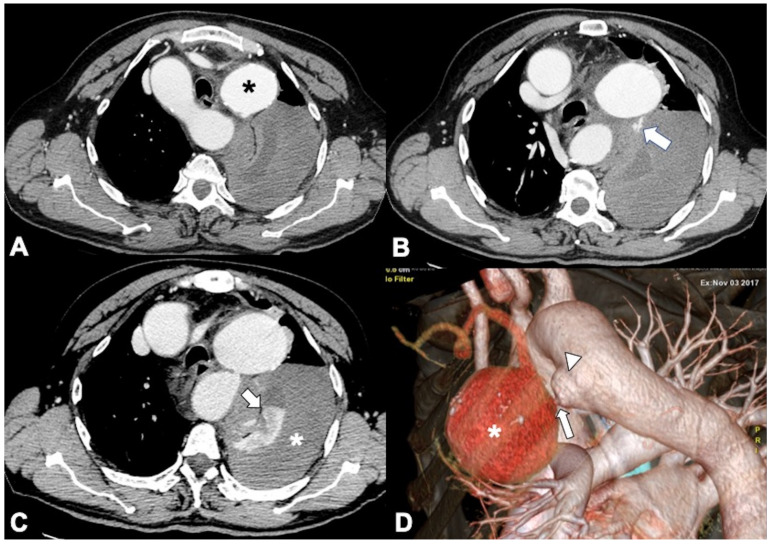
Computed tomography angiography (CTA) provides a rapid noninvasive diagnosis of the entire aorta including aberrant anatomy and pathology. (A) A 74-year-old man presenting in ED with 10 h of abrupt onset of acute chest pain, improving anemia, hypotension (blood pressure of 70/40 mm Hg in both upper extremities), and shortness of breath. CTA shows a right aortic arch and a pleural and extrapleural left hematoma from an aneurysm (asterisk) of a retroesophageal aberrant left subclavian artery (ALSA). (B) More caudal axial scan shows the ALSA aneurysm rupture site (arrow). Note the anomalous course of the left brachiocefalic vein posterior to the ascending aorta. (C) CTA-delayed phase shows contrast medium extravasation in the extrapleural hematoma (asterisk); note extrapleural fat sign (arrow). (D) 3D volume rendering oblique sagittal reformat image clearly shows the aneurysm (asterisk) coming immediately off the ALSA origin (arrow) from a Kommerell’s diverticulum (arrowhead).
